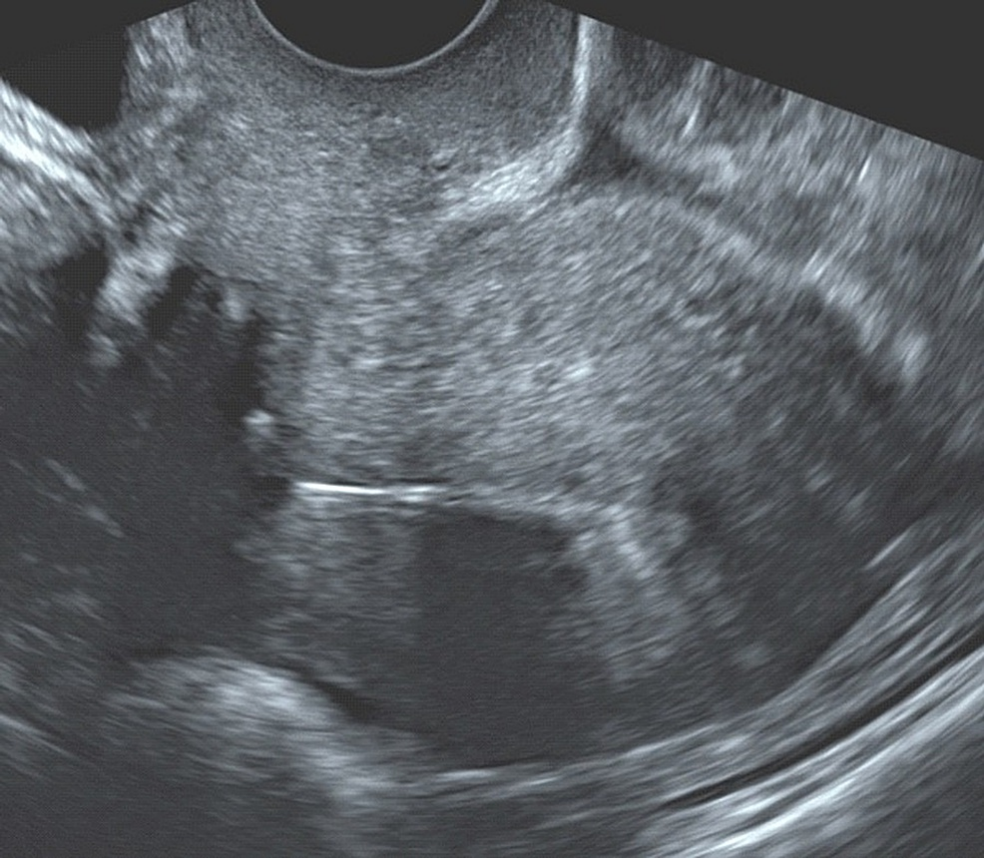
Transvaginal ultrasound showing an anteverted uterus with the Mirena-IUD correctly positioned at the fundus of the uterine cavity
